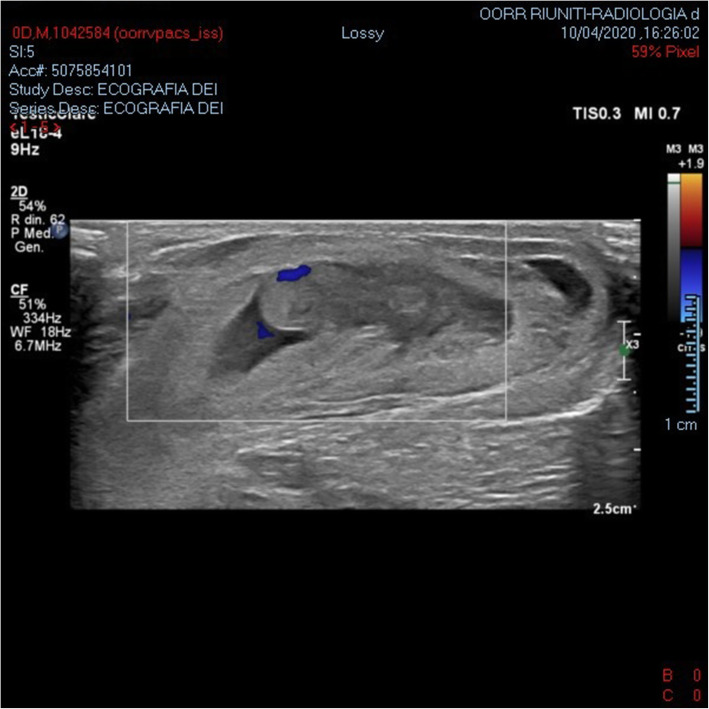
Clear vascular spots are seen on the Echo color Doppler image
